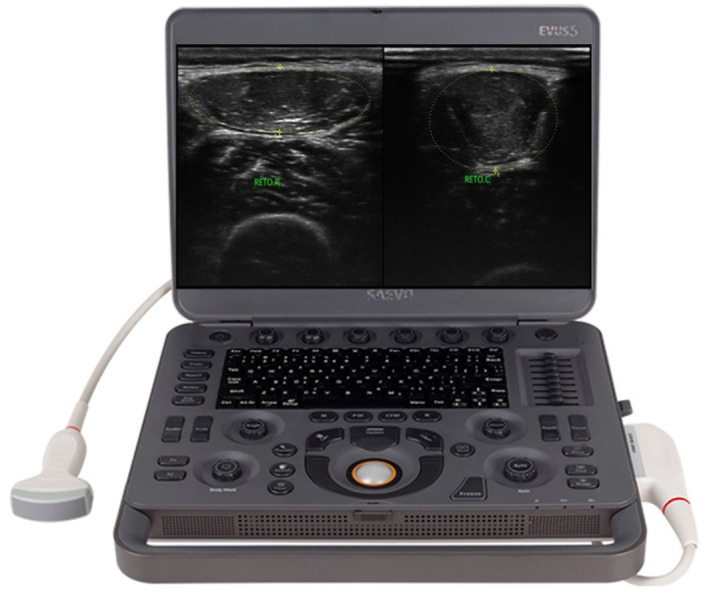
Ultrasound equipment used—SAEVO EVUS5.
